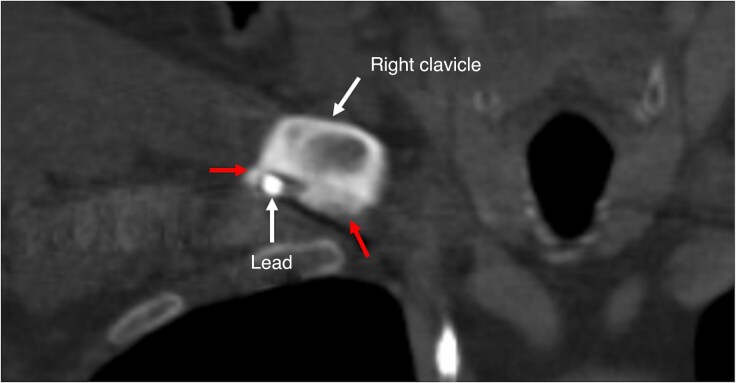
Computed tomography findings in the right costoclavicular space. The lead is in contact with the bone with calcifications (red arrows).
